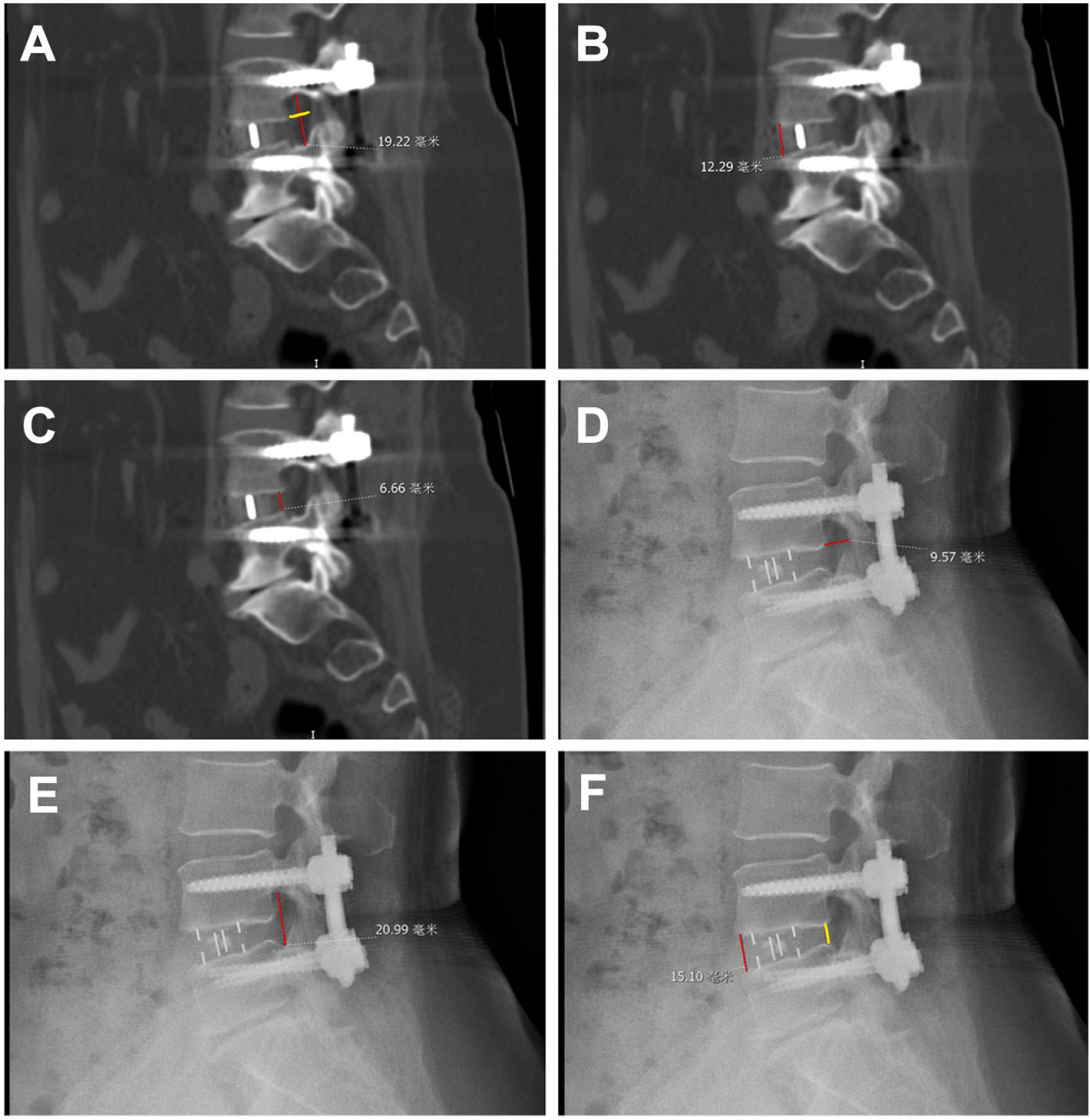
Radiological measurements (CT and X ray) used in this study. a Sagittal computed tomography (CT) image of lumbar vertebrae. The red line shows the foraminal height (FH). The yellow line shows the foraminal width (FW). b Sagittal CT image of lumbar vertebrae. The red line shows the anterior disc height (ADH), (c) Sagittal CT image of lumbar vertebrae. The red line shows the posterior disc height (PDH), (d) Lateral X-ray image of lumbar vertebrae. The red line shows the FW. e Lateral X-ray image of lumbar vertebrae. The red line shows the FH. f Lateral X-ray image of lumbar vertebrae. The red line shows the ADH. The yellow line shows the PDH
